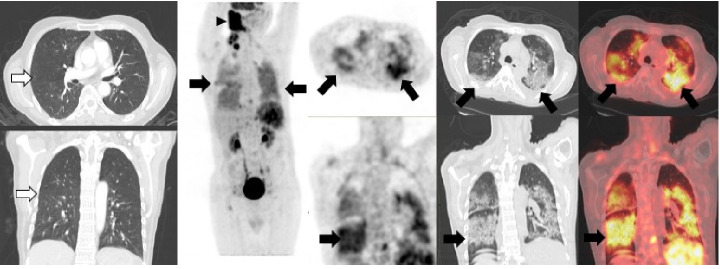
Tomografia de tórax de alta resolución previa a la PET/CT F18-FDG en la que no se evidenció ningún tipo de alteración parenquimatosa (flechas blancas). En la PET/CT F18-FDG se evidenció una masa hipermetabólica intensa, SUVmáx de 14, en la región cervical derecha y áreas de ulceración cutánea, conglomerados adenopáticos supraclaviculares derechos y múltiples focos hipermetabólicos infiltrativos en el parénquima esplénico relacionados con compromiso por linfoma (cabeza de flechas negras). En el tórax (flechas negras) se detectaron derrames pleurales bilaterales sin actividad metabólica y múltiples opacidades alveolares hipermetabólicas, así como un patrón de vidrio esmerilado de distribución en parches de carácter multilobar en ambos parénquimas pulmonares, con SUVmáx de 4,5.
